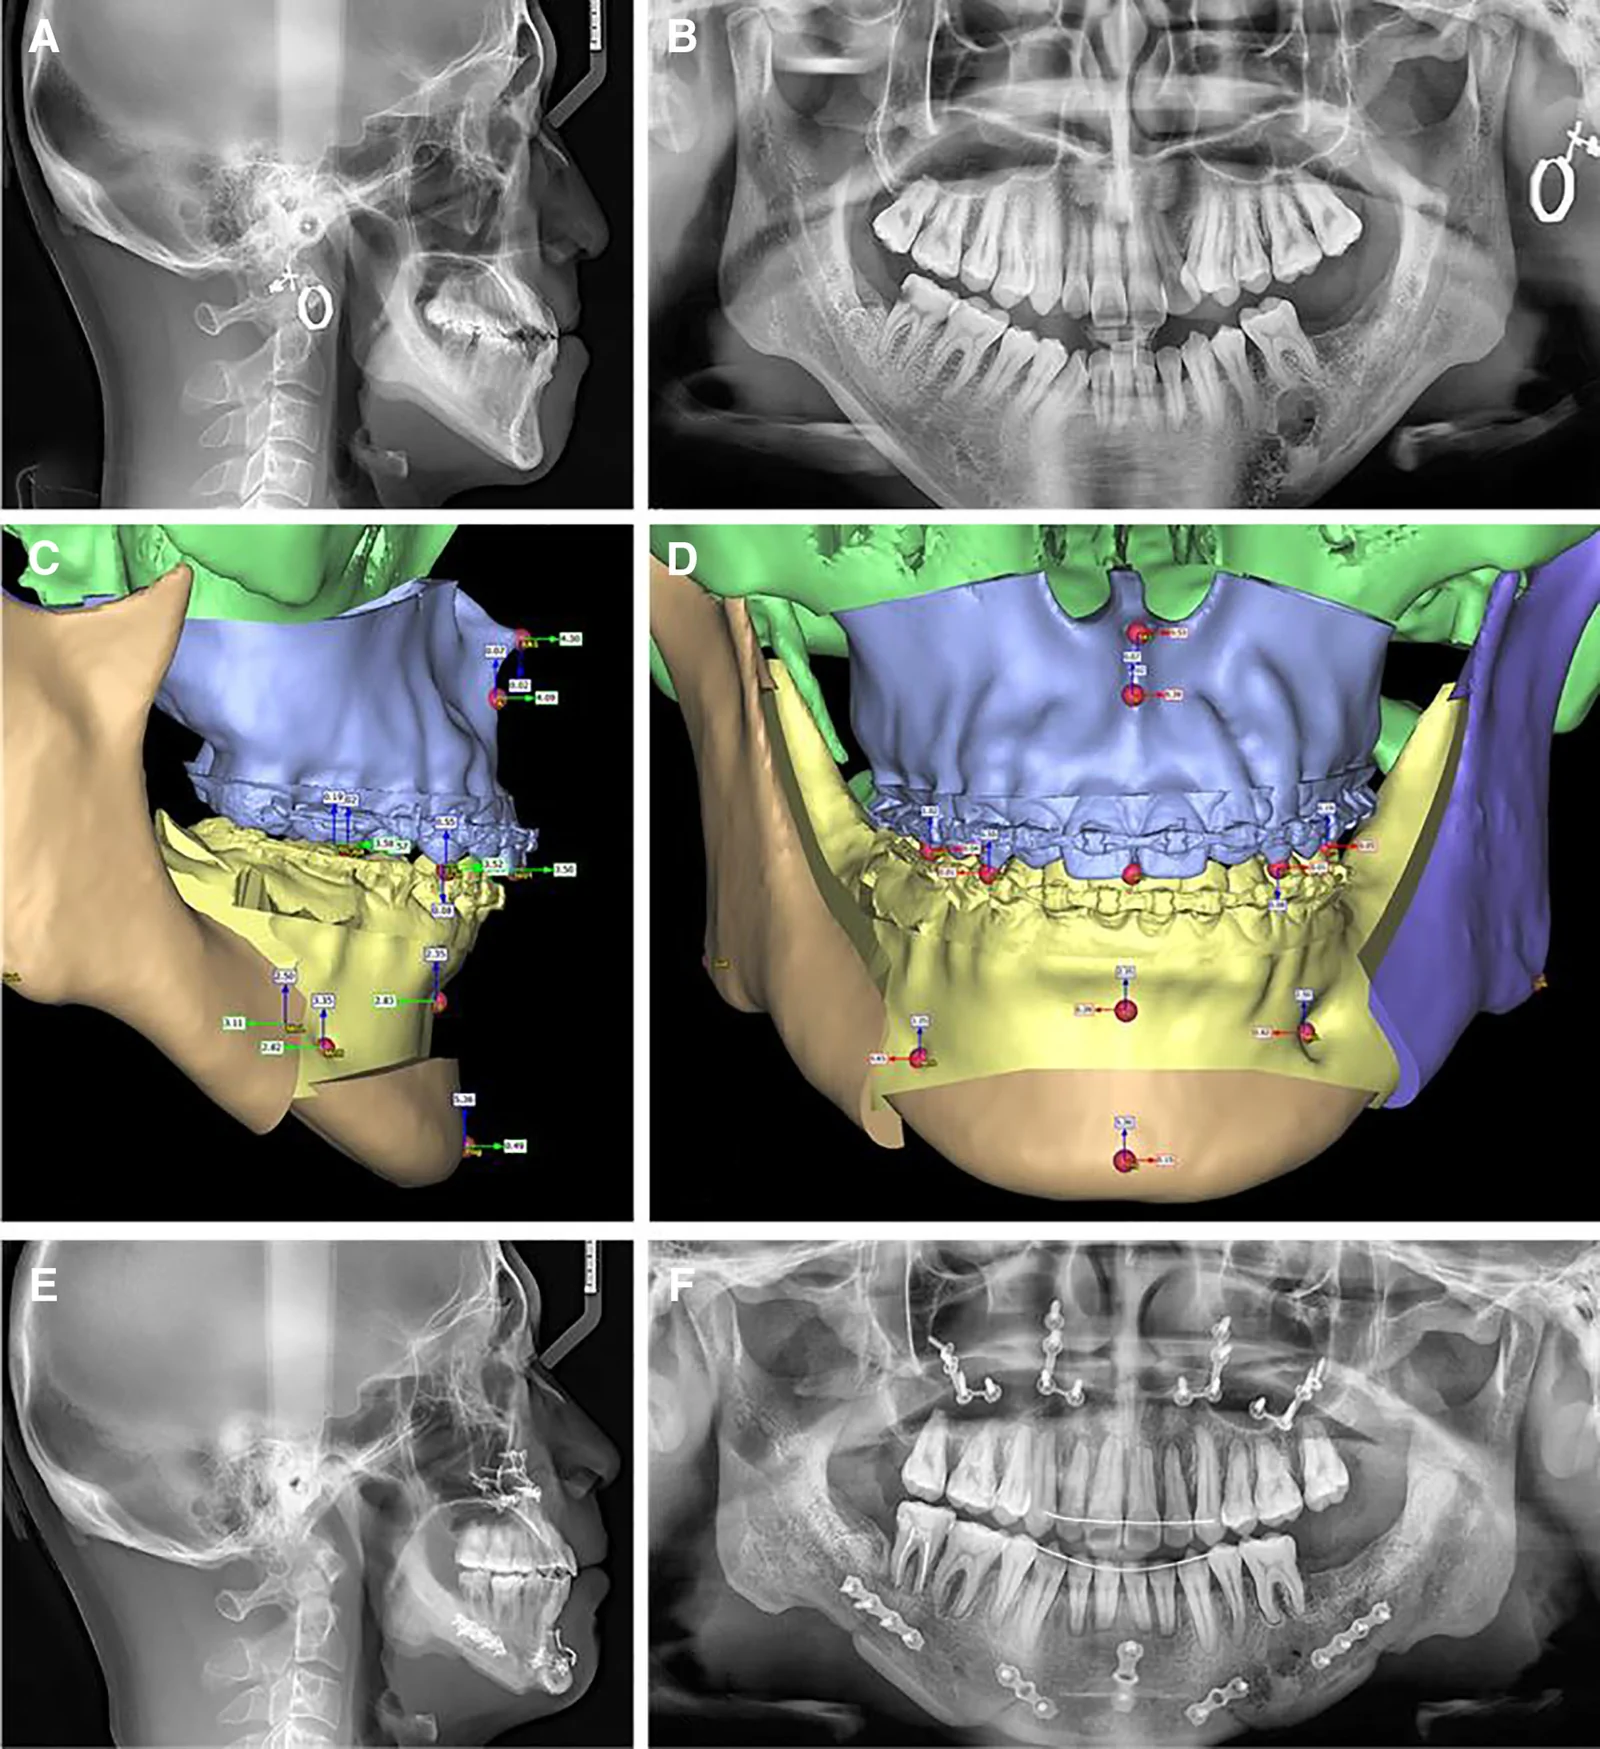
Case 3, radiographs. (A, B) Pretreatment. (C, D) Surgical plan. (E, F) Posttreatment.
